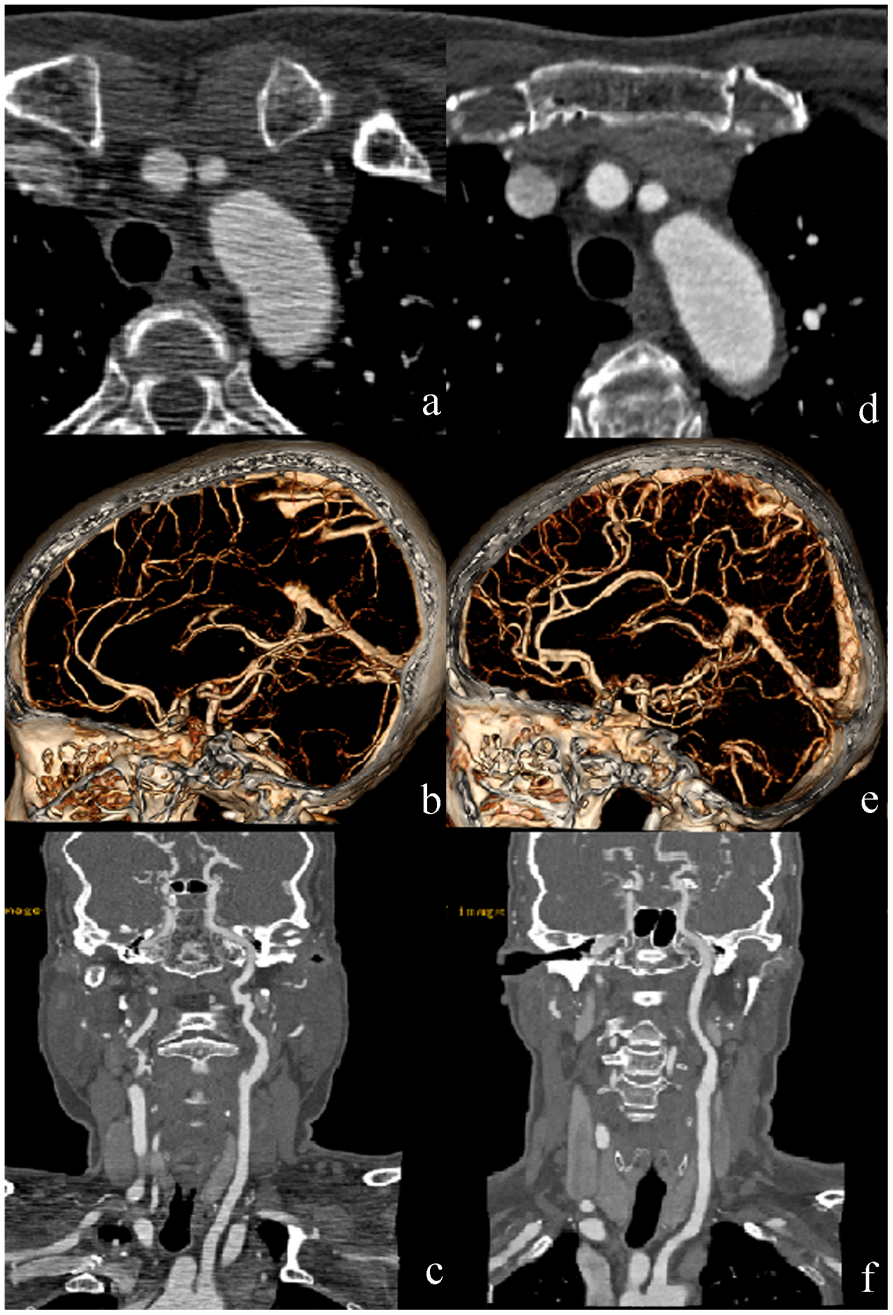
The images compared between two protocols. (a–c) CT images in a 64-year-old woman (BM, 62 kg; BMI, 26.84) in protocol A. (d–f) CT images in another 64-year-old woman (BM, 68 kg; BMI, 26.56) in protocol B. (a) and (d) axial images; (b) and (e) volume rendered images; (c) and (f) curved planar reconstructed images. The images in protocol B with iterative reconstruction decreased the streak artifacts and noises without losing the vessel sharpness and image quality.
